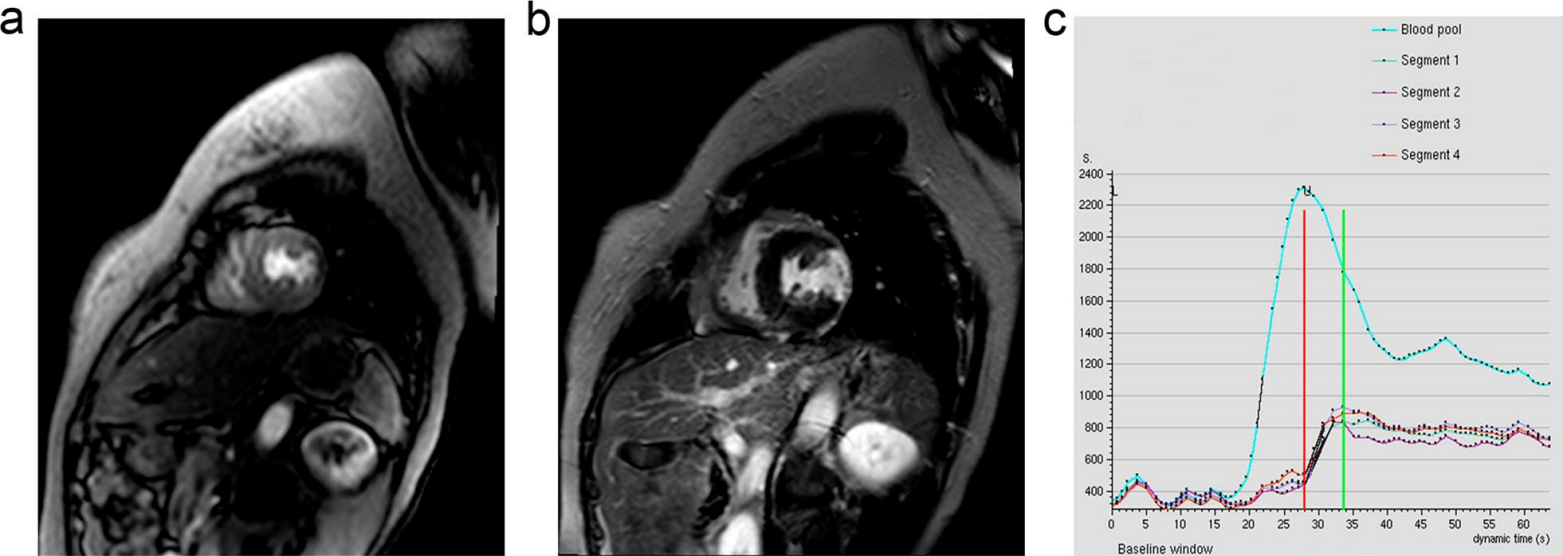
Representative images of cardiac magnetic resonance (CMR) in the 48-year-old female patient with MINOCA. a Myocardial first-pass perfusion magnetic resonance imaging indicated apical perfusion defect of left ventricular. b Late gadolinium enhancement (LGE) imaging indicated that lateral wall of apical cap (16th segment) occurred the transmural myocardial infarction, and it was supplied by LCX coronary artery. Besides, the transmural extent was grade IV, and area of myocardial infarction was 17.8%. c Signal intensity curves indicated that signal intensity of 16th segment decreased at the apical cap of left ventricular apex
